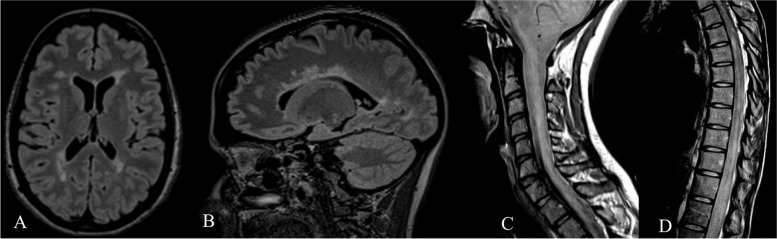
MRI of case 3 following alemtuzumab and subsequent relapse. A & B Brain imaging (axial and sagittal FLAIR sequences, respectively) with periventricular and subcortical lesions. C Cervical cord imaging (sagittal proton density-weighted sequence) with diffuse longitudinally extensive hyperintense signal throughout the cervical cord. D Thoracic cord imaging (sagittal proton density-weighted sequence) with multifocal cord lesions
